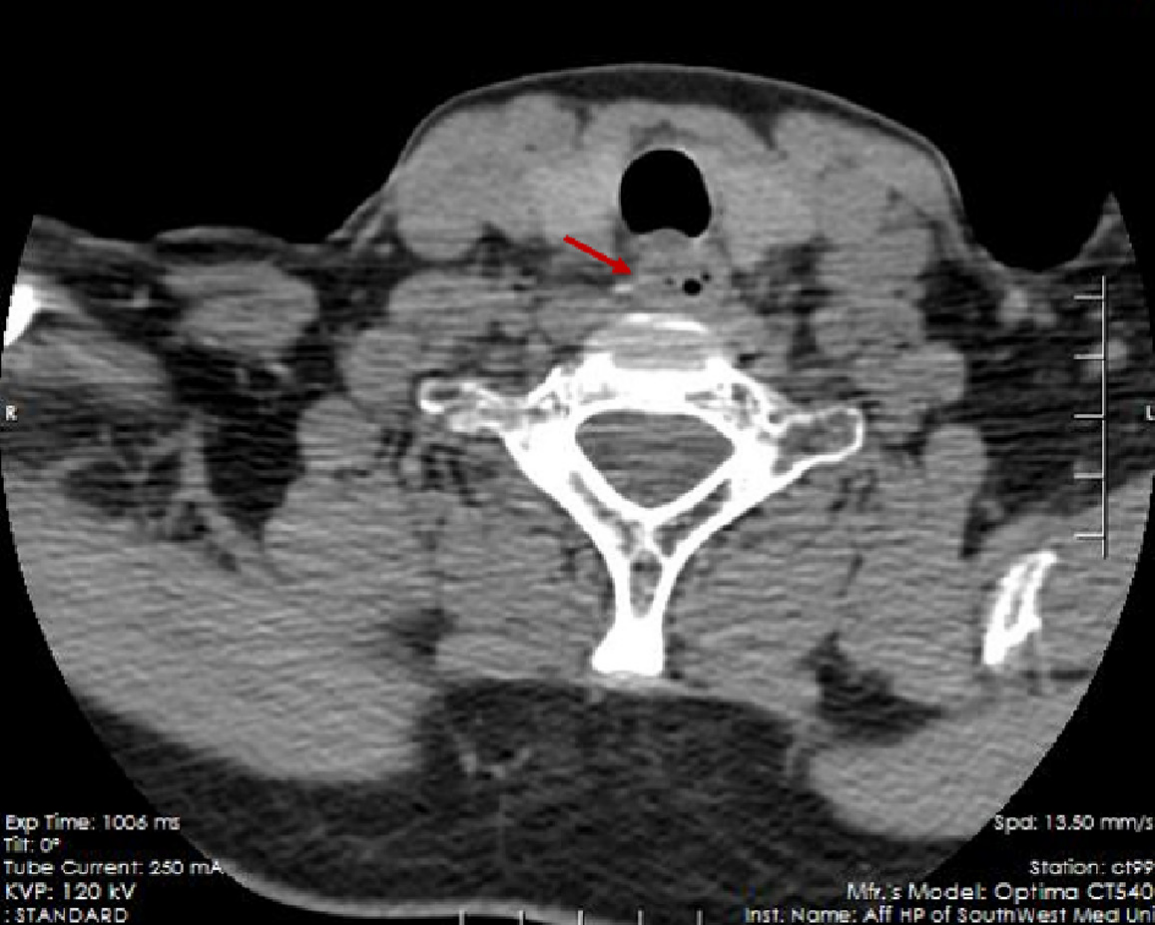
Computed tomography showed a dense linear shadow penetrating the anterior wall of the proximal esophagus (red arrow).
